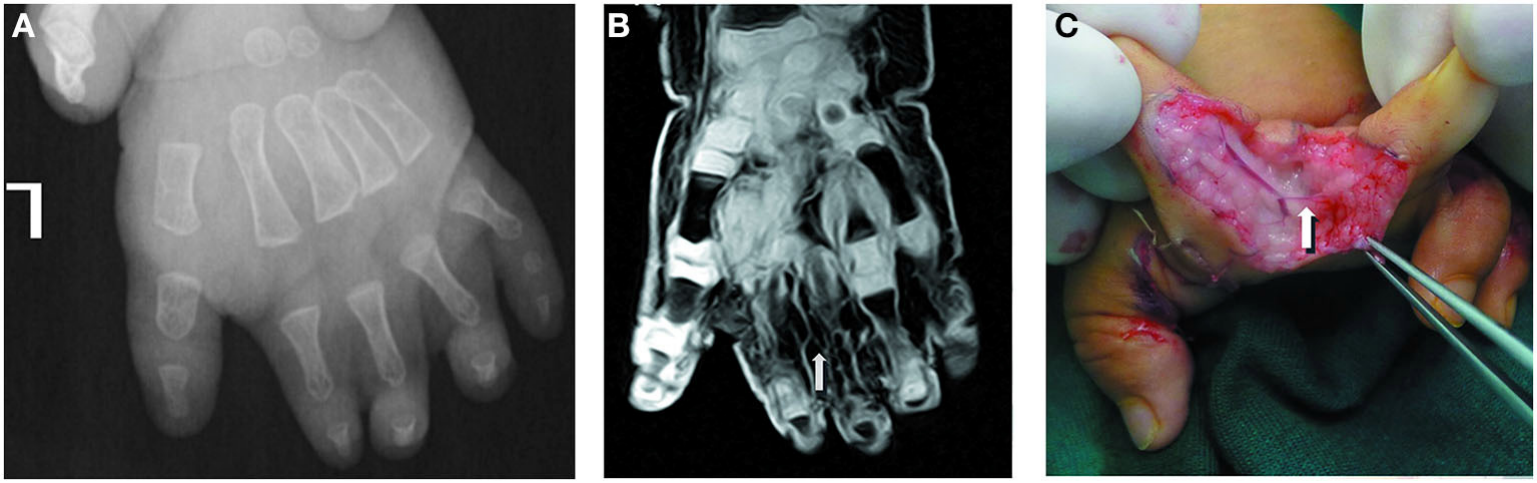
A 13-month-old male patient with complicated syndactyly of the left 1st-2nd-3rd-4th-5th digits. (A) The preoperative radiograph showed the fused extent of proximal soft tissues of the 1st-2nd-3rd-4th-5th webspaces and only two phalanges of the 2nd-3rd-4th digits. (B) A contrast-enhanced three-dimensional water selective cartilage scan indicated that the proper palmar digital artery of the radial side of the 3rd finger (white arrow) was thin. (C) This was completely consistent with the intraoperative observation results.
